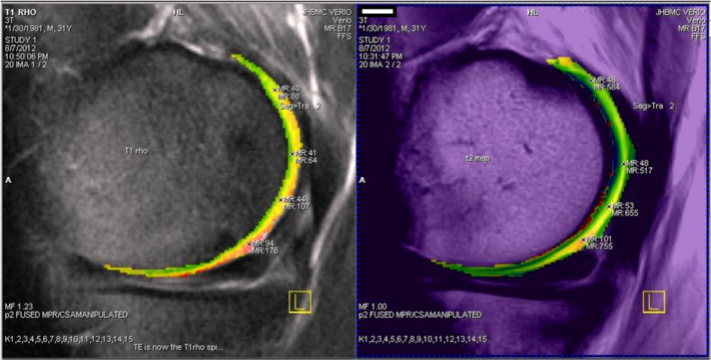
Magnetic resonance imaging and biochemical imaging of normal articular
